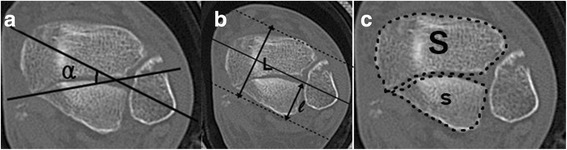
The radiographicmeasurements on the cross section. a The cross angle (α) wasdetermined by measuring theangle between the bimalleolaraxis and the major fracture line. b The fragment lengthratio (FLR) was determined by calculatingthe percent of length (l)/length (L). c The fragment arearatio (FAR) was determined bycalculating the percent of area(s)/area (s + S)
